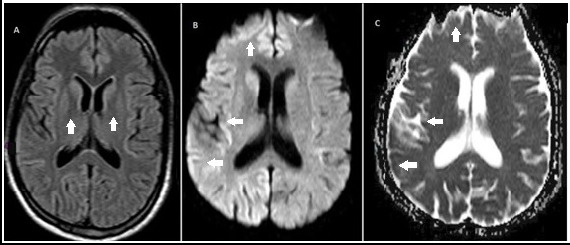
Brain MRI. Panel A show hyperintensity in bilateral striatum in the FLAIR sequence. Panels B and C shows water restriction in cortex with a predominance in the right hemisphere (DWi/ADC map).
